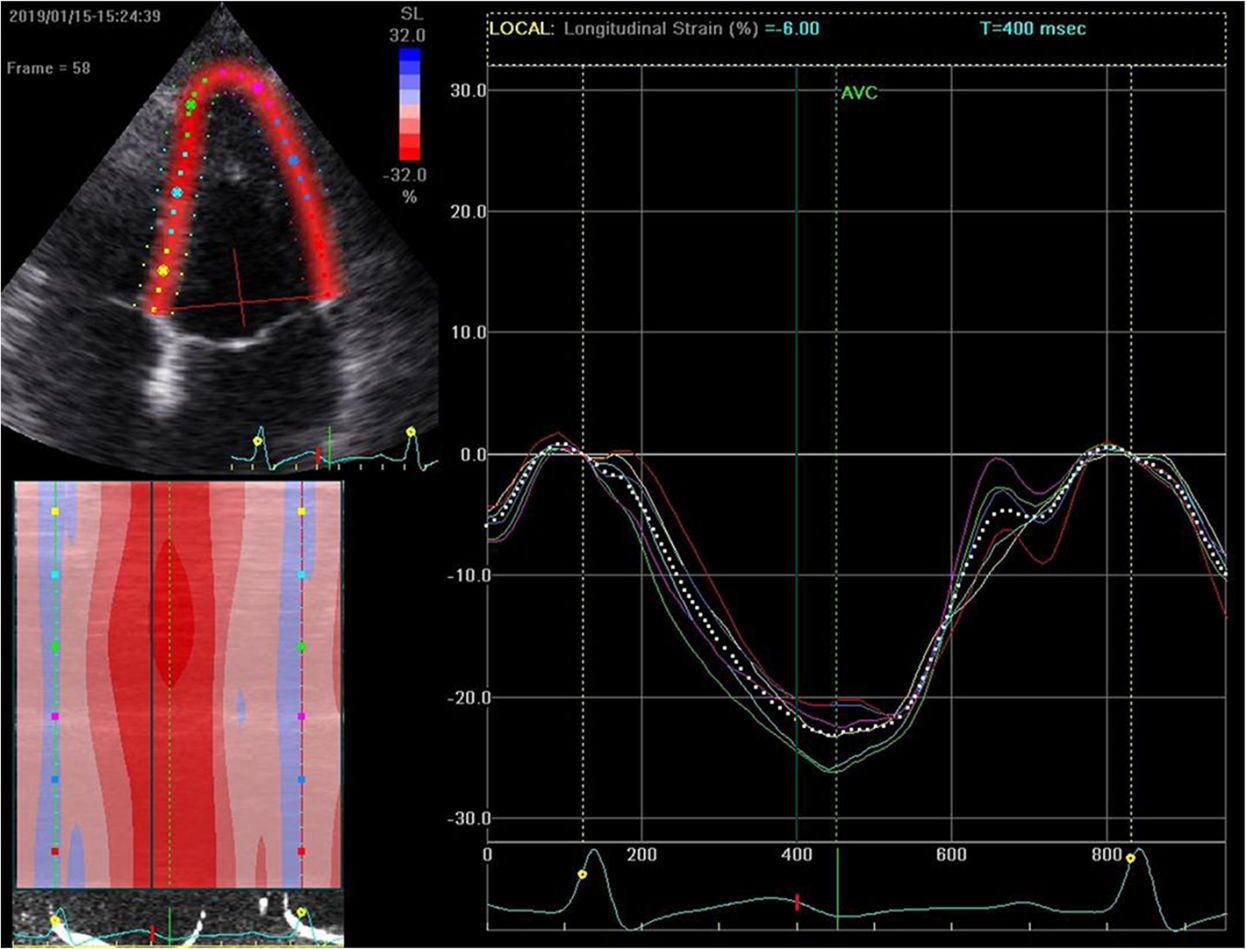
Measurement of GLS of a healthy individual by 2D-STE. The image was obtained from the apical four-chamber view
